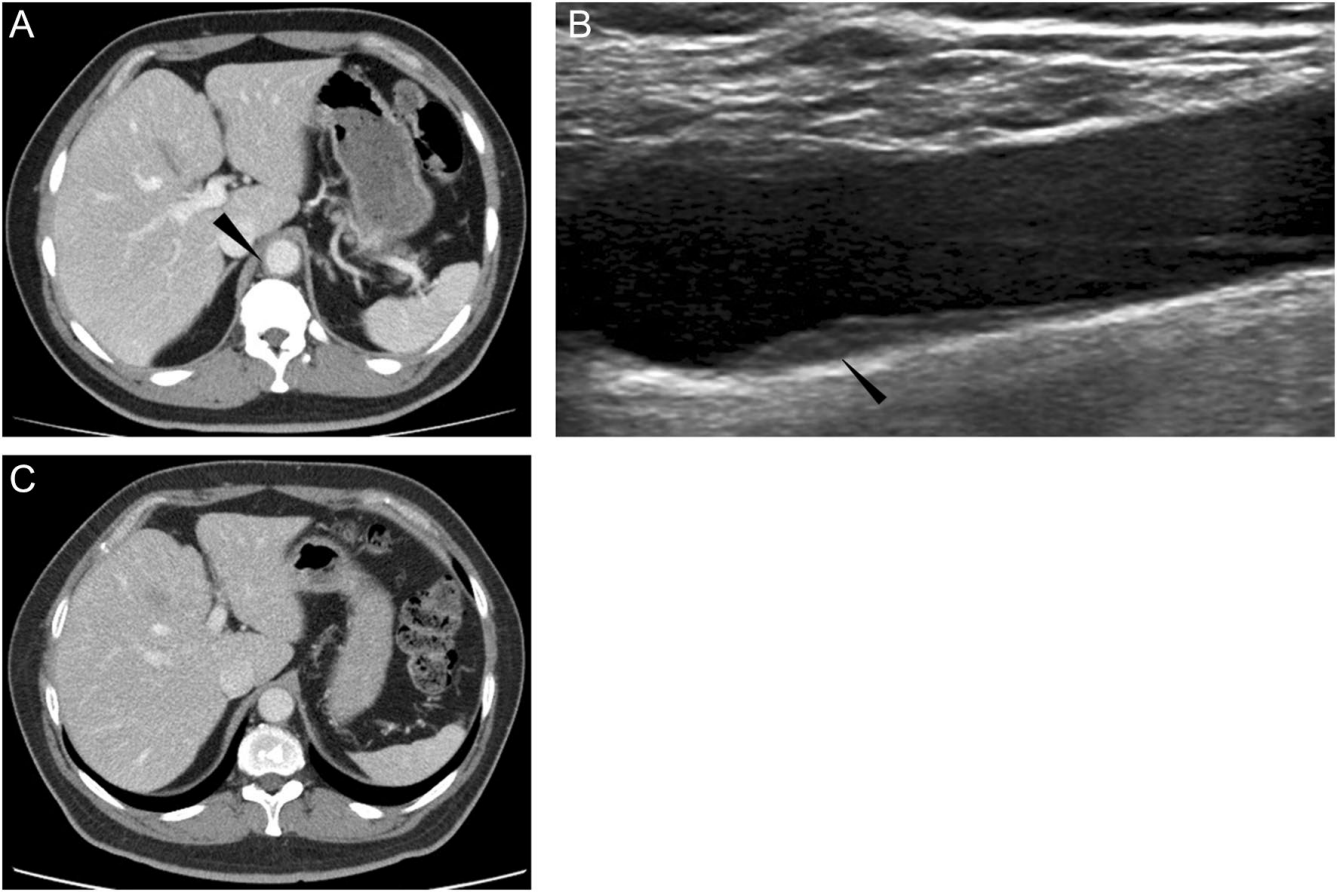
The image findings of the vascular lesions. A: abdominal contrast-enhanced computed tomography (CT) imaging at diagnosis. The aortic wall is thickened (arrow). B: carotid ultrasonography before treatment. The carotid artery thickened (arrow). C: abdominal contrast-enhanced CT imaging after treatment. Aortic wall thickening improved
